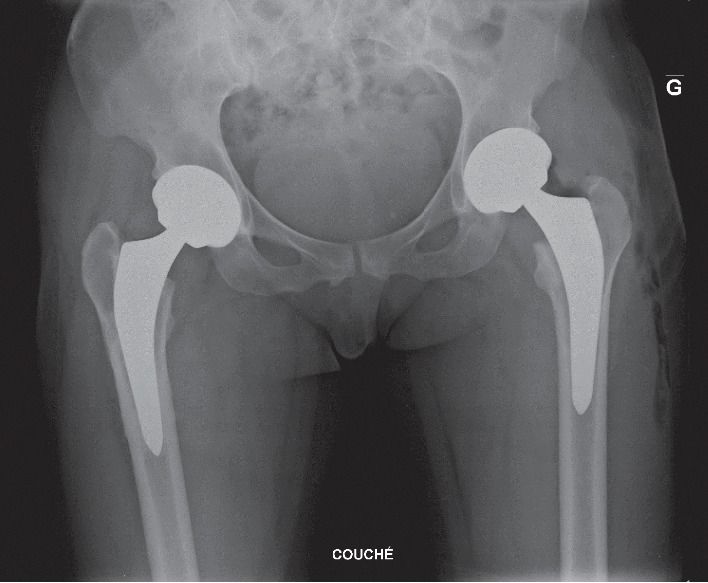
Postoperative pelvic X-ray after left total hip arthroplasty and three years after right total hip arthroplasty. Implants are in good position.
